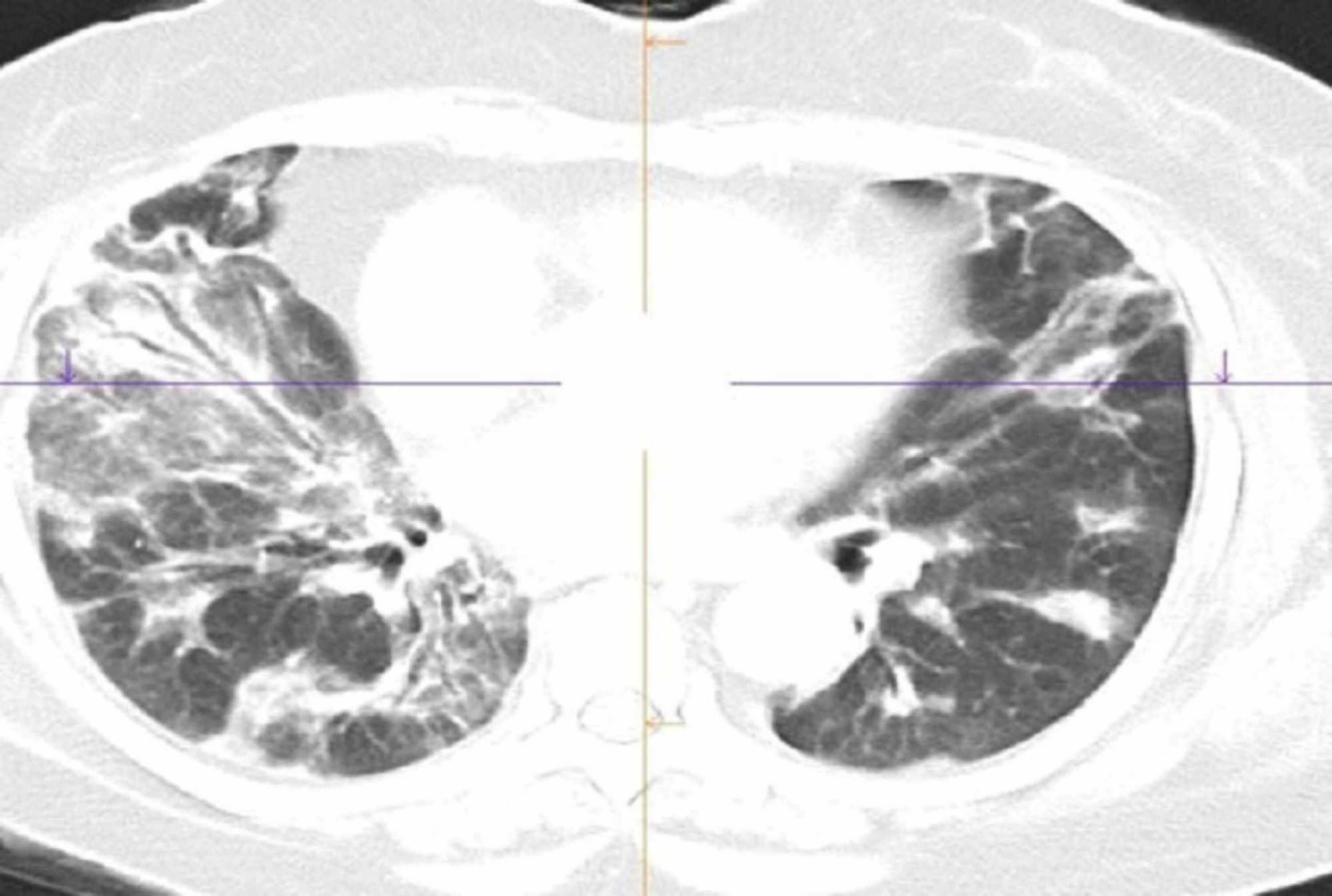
MDCT axial view of thorax showing bilateral ground glass attenuation with areas of consolidation MDCT: Multidetector computed tomography
